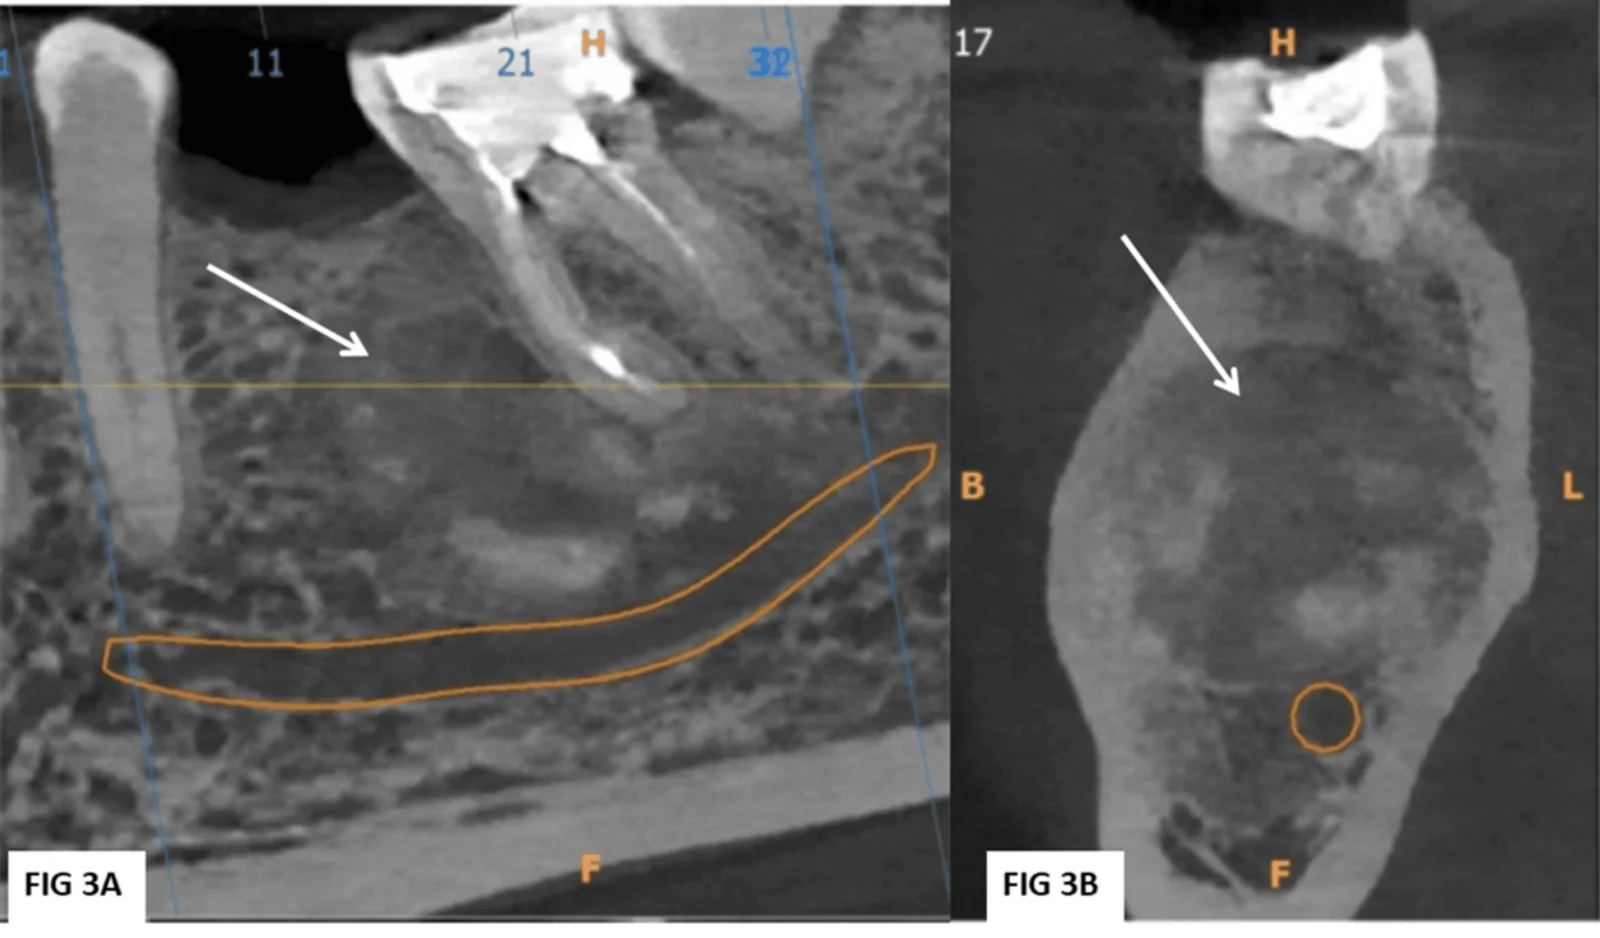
Cone beam computed tomography (CBCT) images at six-month follow-up (A) The arrow indicates the grafted site, which shows radio-opacity and new bone formation in the panoramic view. (B) The arrow indicates the grafted site, which shows radio-opacity and new bone formation in the sagittal view.
